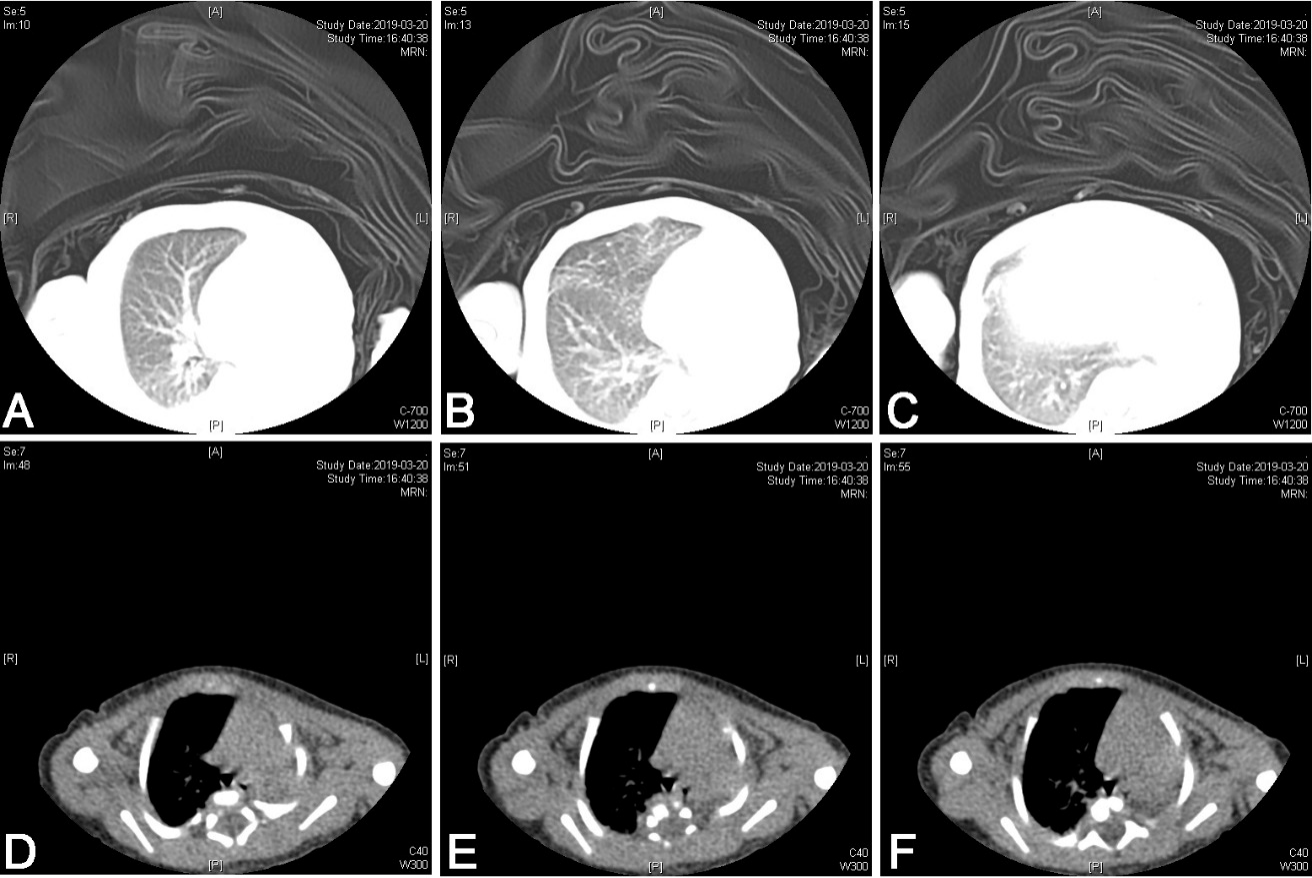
Thoracic CT indicated that the left lung and the primary bronchus of left lung were not available in pulmonary window. No shadows with aberrant densities were noticed in the right lung (A–C). In addition, there was mediastinal shift to left side in mediastinal window. The structures and boundaries in left mediastinum were not completely displayed (D–F).
